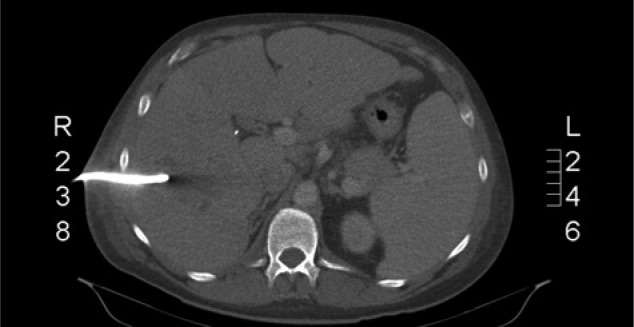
Computed tomography–guided drain placement of pyogenic liver abscess with. A catheter was placed in the space-occupying lesion seen above by ultrasound and magnetic resonance cholangiopancreatography. This yielded purulent fluid. A drain was placed, which can be seen traversing the abdominal wall in the region occupied by the pyogenic liver abscess.
